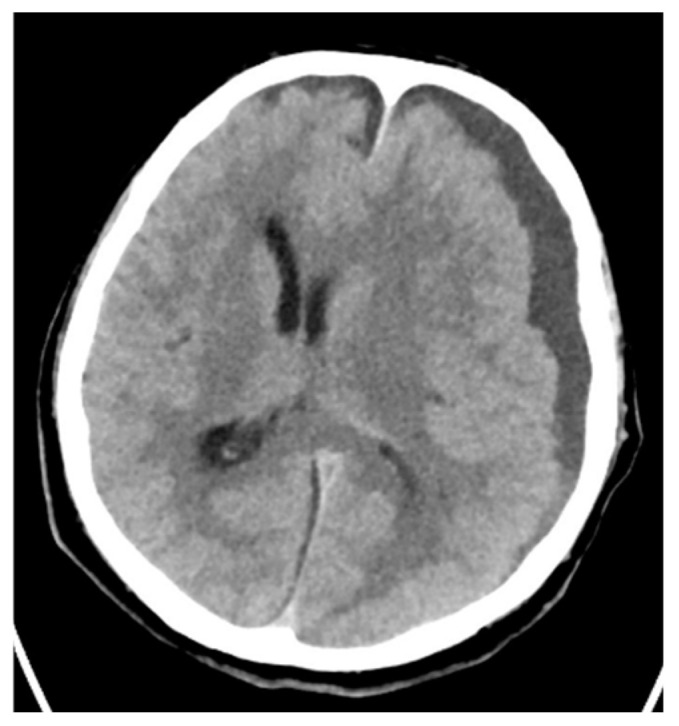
Brain CT. Cerebral abscess caused by *S. pyogenes*.
